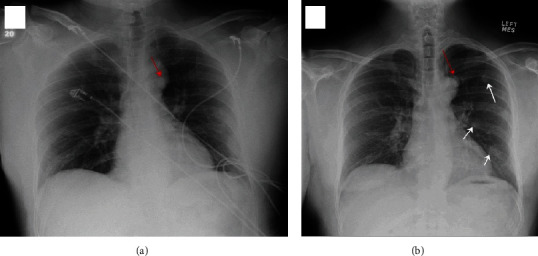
Chest X-ray. (a) Anteroposterior view showing a 1.7x1.4 cm nodular density at the level of aortic arch (red arrow). No other definitive nodules were seen. (b) Posteroanterior radiograph six months later, revealing the previously noted nodular density (red arrow) that remains unchanged and several new nodular opacities (white arrows) in the left upper and left lower lung fields.
